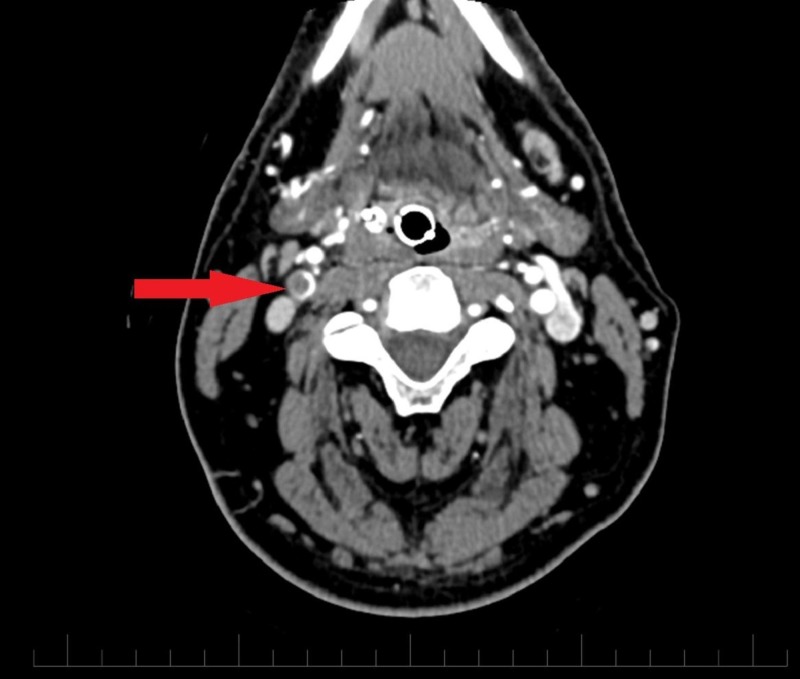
CT angiogram neck (transverse section) showing nonocclusive thrombus in the right internal carotid artery CTA neck demonstrates a hypodense filling defect (red arrow) in the right internal carotid artery suggestive of nonocclusive thrombus CTA: computed tomography angiogram
